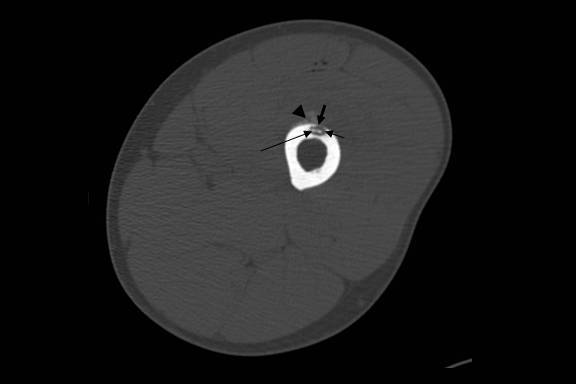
CT image in bone window showing a lucent cortical lesion. Retrospectively, the central calcification (long thin arrow) and the inner margin of the nidus (short thin arrow) were irregular. There was periosteal reaction (arrow head) with a small cortical break (short fat arrow).
